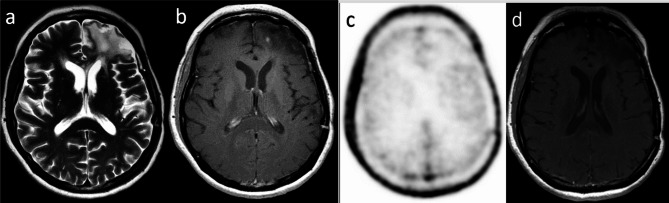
[^18^F]FLT-PET/MRI in a patient with low grade glioma after complex treatment (partial resection, chemotherapy and radiotherapy). In the area of post-treatment changes (a) in the left frontal lobe, two years after the last treatment, there was a new spot of contrast enhancement (b) without increased PET activity (c). This enhancement spontaneously regressed six months later, and this lesion was reported as post-treatment changes. CeMRI in this case was false positive
